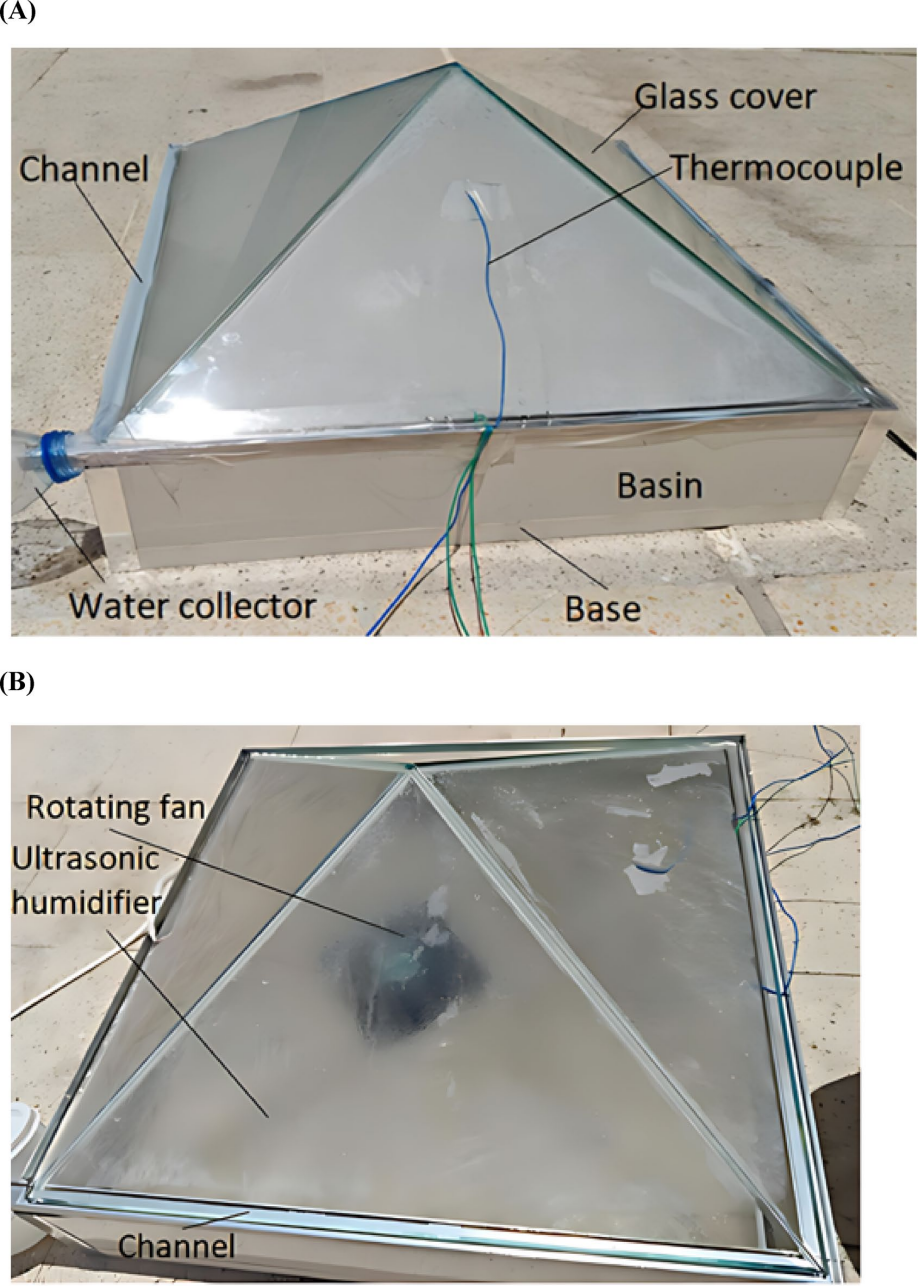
(A). Experimental test risk. (B). Solar still shows the rotating fan and ultrasonic humidifier.
